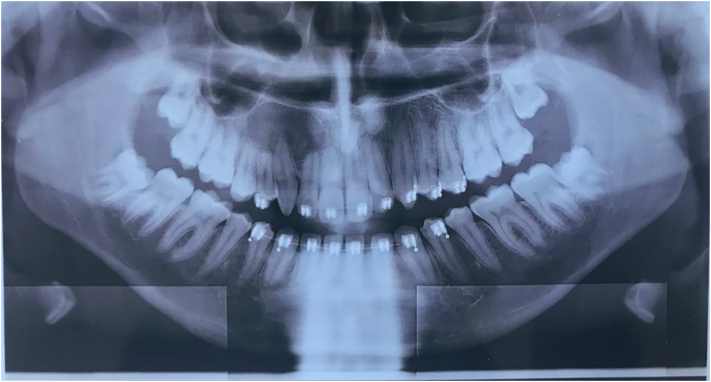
Panoramic radiograph showing the beginning of bone regeneration of the area, 9 months after surgery.
